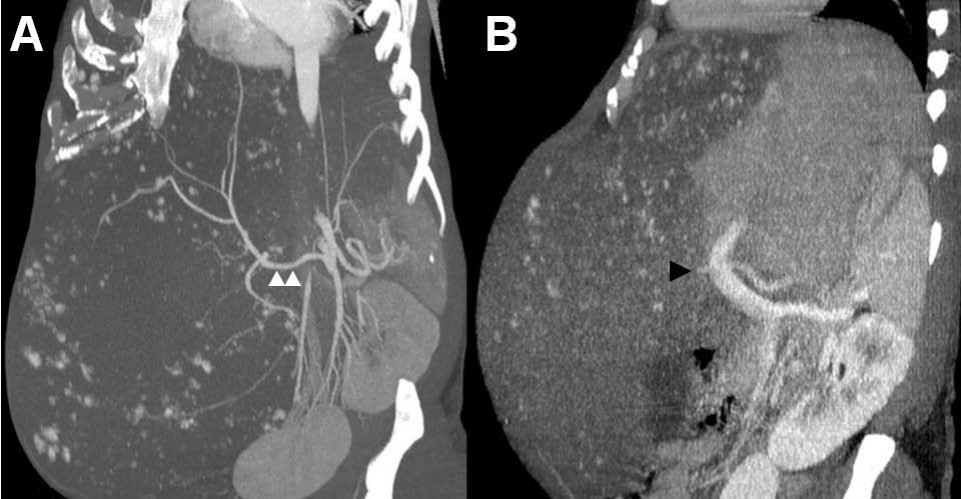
Vascular reconstruction images. (A) Angiography images reconstructed by multi-detector computed tomography (MDCT). The right hepatic artery (white arrowheads) and its branches are markedly stretched. (B) Portography images reconstructed by MDCT. The right portal vein is occluded (black arrowhead).
